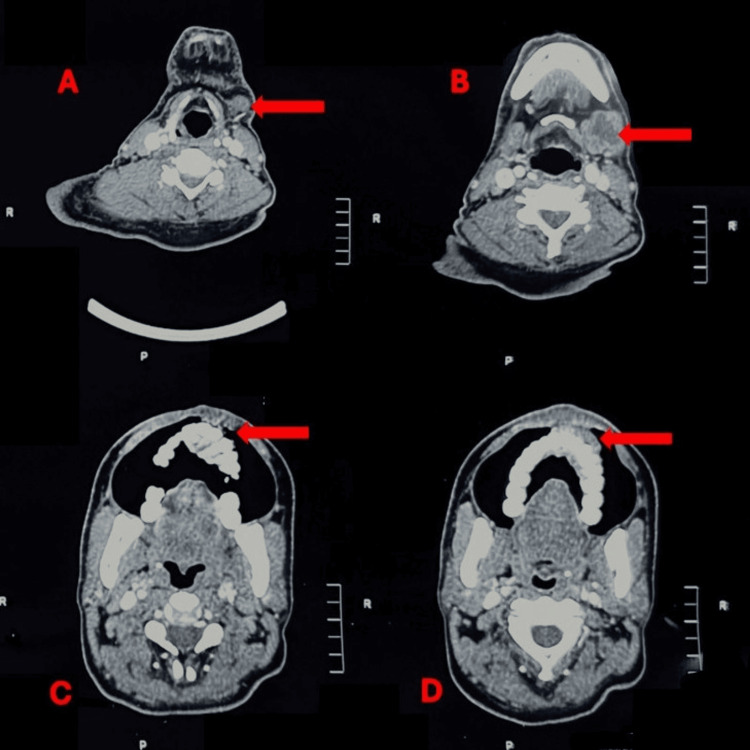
Post-immunotherapy contrast-enhanced computed tomography scan (CECT) of head and neck (axial view) (A, B) Multiple enlarged cervical lymph nodes with loss of fatty hilum and areas of central necrosis (red arrows). (C, D) Infiltrative soft tissue mass involving the hard palate with erosion of the adjacent maxillary bone and extension into the gingivobuccal sulcus (red arrows).
